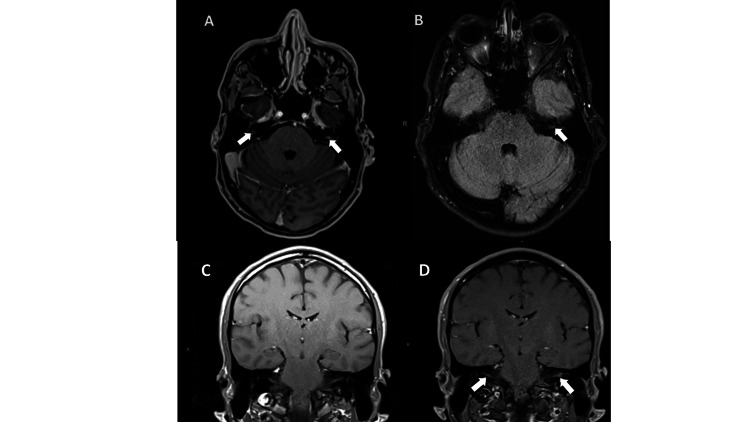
Axial post-contrast T1 (A), axial FLAIR (B), coronal pre-contrast T1 (C), coronal post-contrast T1, and (D) brain magnetic resonance imaging The image shows enhanced linear areas within the internal auditory canals bilaterally, with the left side exhibiting more enhancement than the right, specifically along the intracanalicular segment of the facial nerves, accompanied by a corresponding FLAIR hyperintense signal (white arrow). FLAIR, fluid-attenuated inversion recovery.
